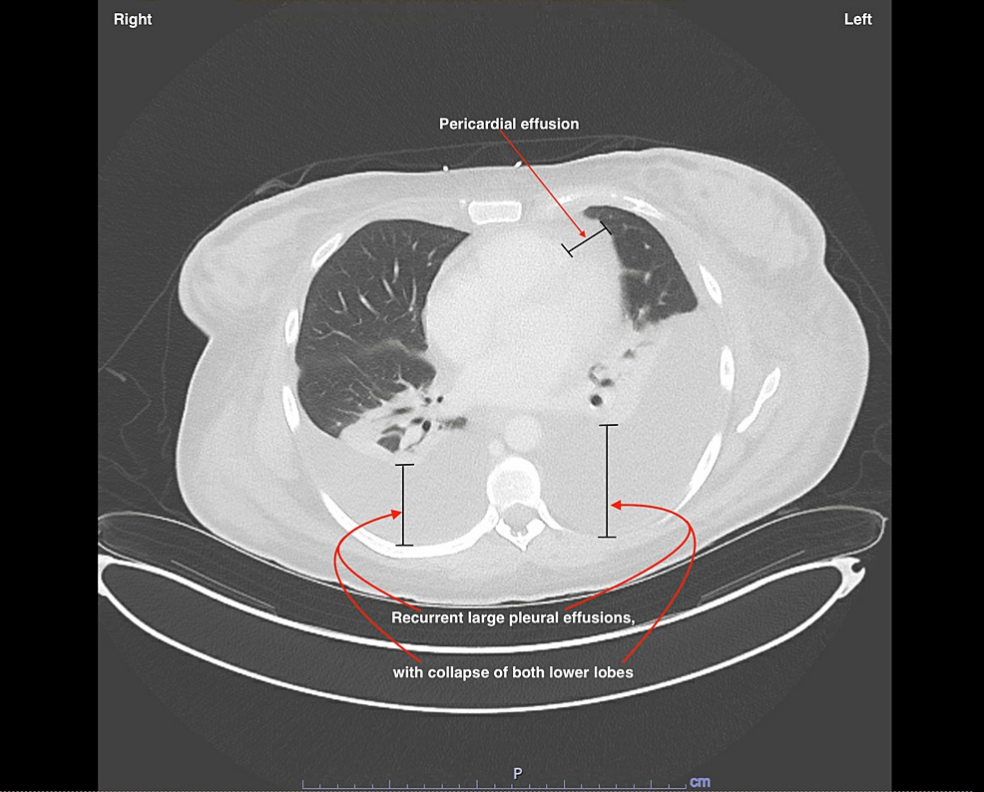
CT chest image The image shows recurrent large pleural effusions with the collapse of both lower lobes and pericardial effusion.
